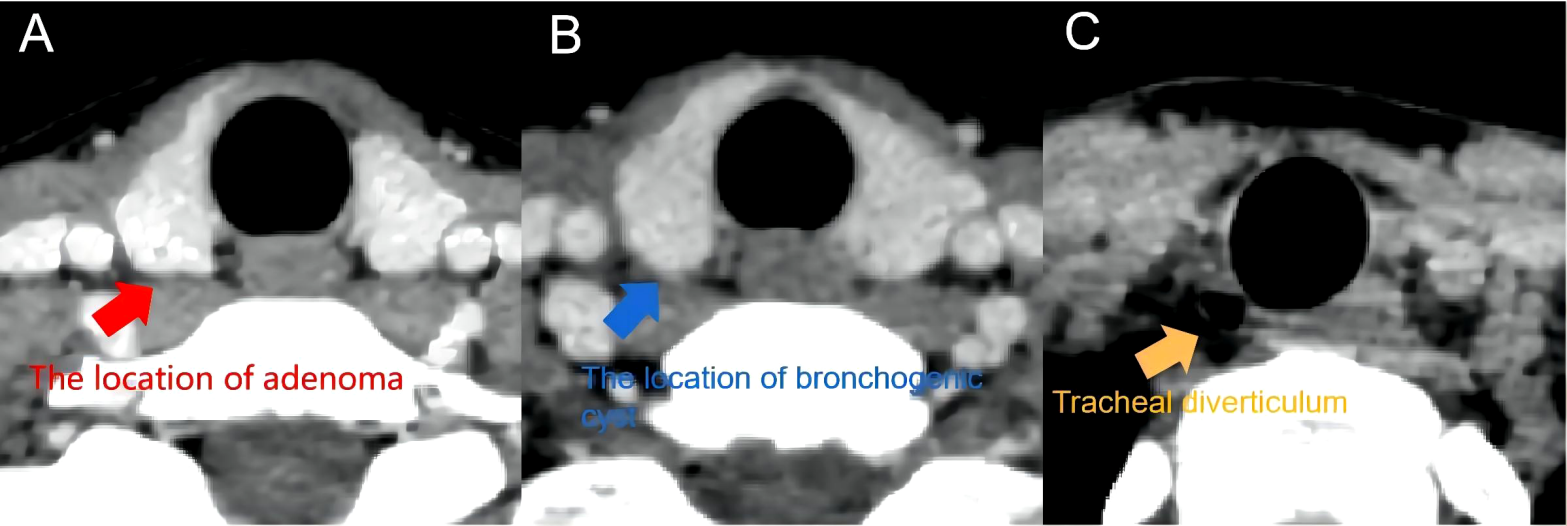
Postoperative CT. (A) the location of adenom in postoperative CT. (B) the location of cervical bronchogenic cyst in postoperative CT. (C) tracheal diverticulum in postoperative CT.
